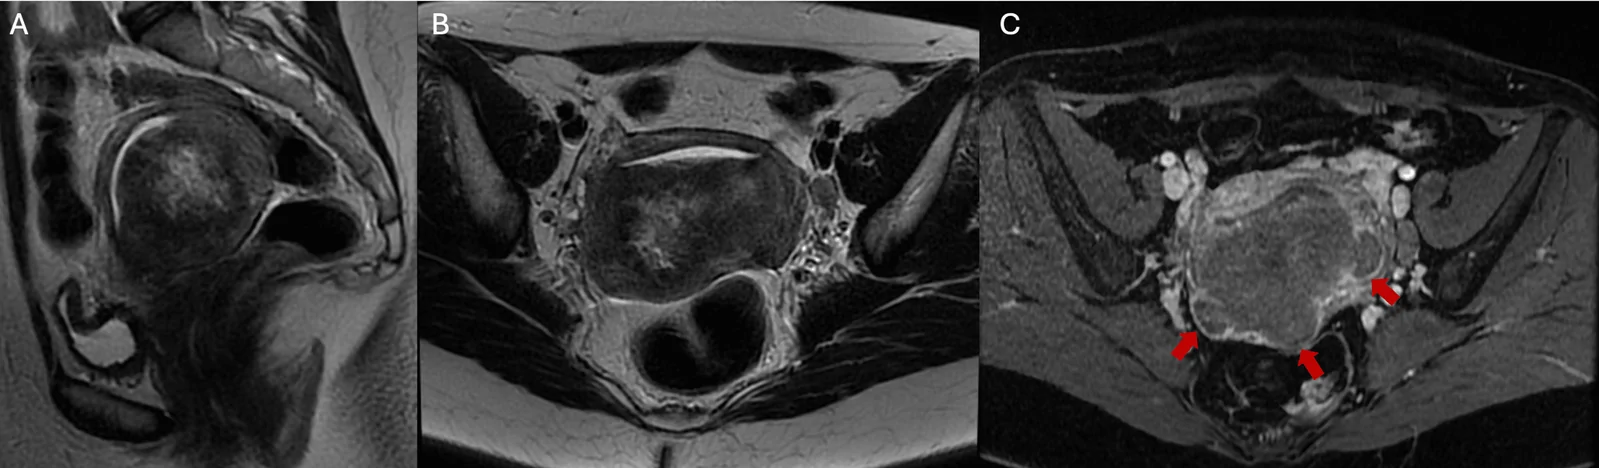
MRI features of the uterine tumour. Sagittal T2-weighted (A) and axial T2-weighted (B) images of the pelvis demonstrate a heterogeneous tumour centred in the posterior uterine body. The axial T1 fat-saturated post-gadolinium image (C) shows the tumour to be hypointense relative to the surrounding myometrium, with invasion of the outer myometrium at three distinct sites (red arrows), without disruption of the serosa.
